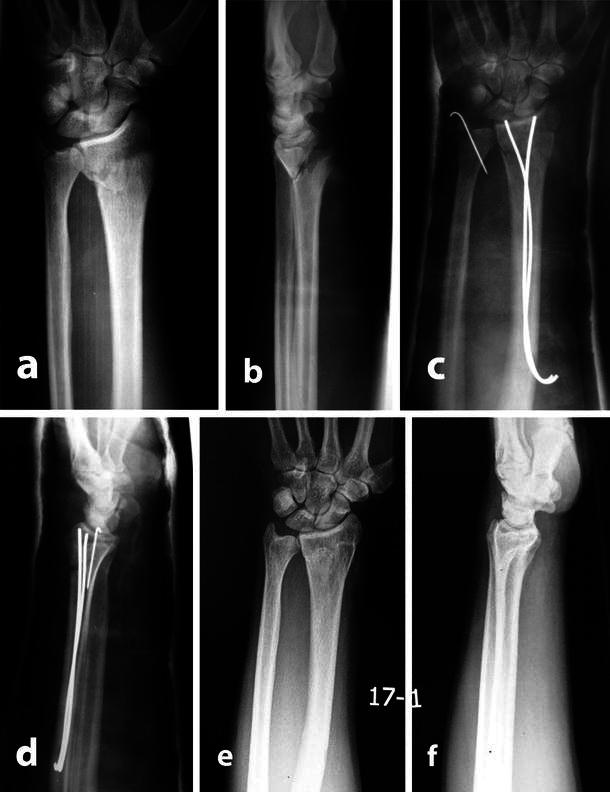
a and b preoperative radiographs of a 37-year-old male with Frykman’s type II (AO; A 2-2) fracture of left radius and fracture of base ulnar styloid; (c and d) post-operative radiographs showing retrograde K-wire used for fixation of ulnar styloid; (e and f) radiographs one and a half years after removal of K-wires showing an excellent radiological score
